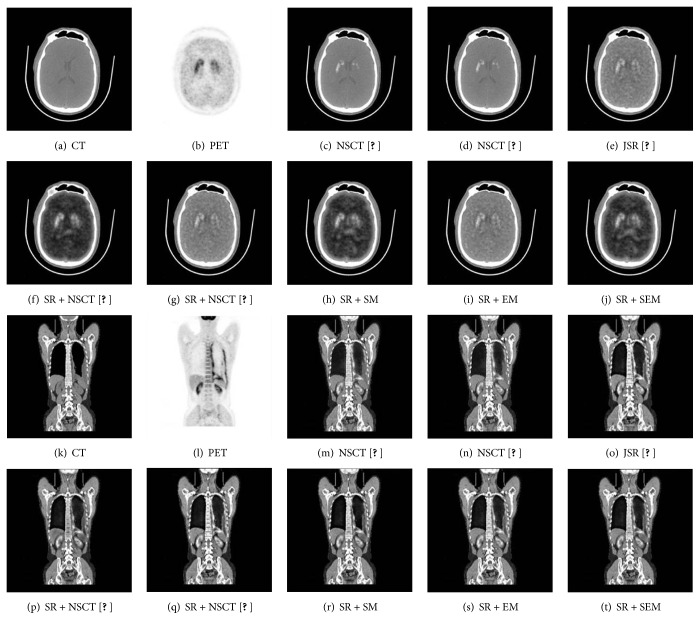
The CT and PET image fused results of different fusion methods.
